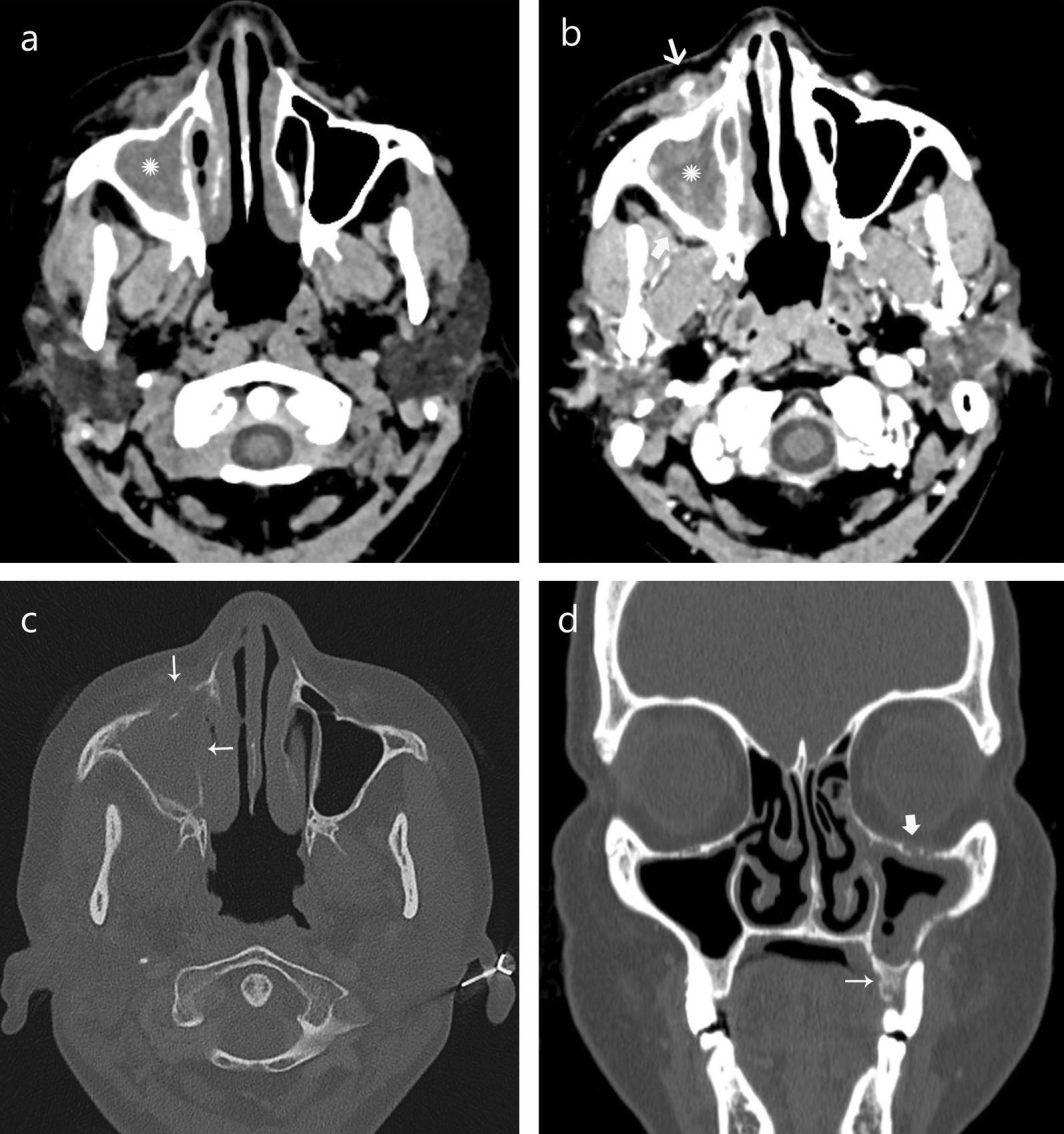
Computed tomography findings in mucormycosis. a Non-contrast and b contrast enhanced CT scan demonstrates hypodense soft tissue obliteration of right maxillary sinus (*) with heterogeneous, patchy mucosal enhancement and enhancing foci in cavity with inflammatory changes in premaxillary (thin arrow), retroantral fat (thick arrow) and facial muscles. c CT scan 20 days later shows extensive erosion of anterior and medial walls of maxillary antrum (arrows). d Coronal view of face shows mucosal thickening of left maxillary and anterior ethmoidal air cells, obliteration of the osteo-meatal complex, erosion of maxillary antrum (superior and lateral wall) and alveolar arch (thin arrow) along gingivo-buccal sulcus against 2^nd^ upper molar. The left infraorbital foramen is thickened suggesting perineural spread along infraorbital nerve (thick arrow)
